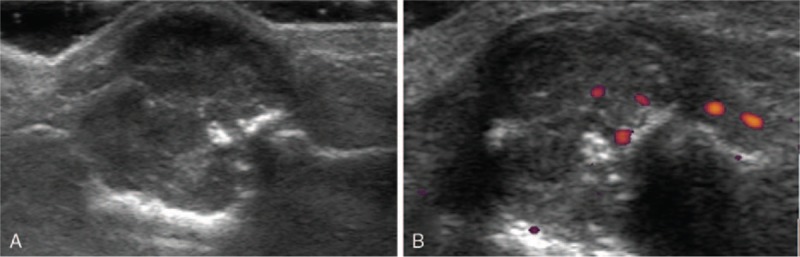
Case 2. (A) Two-dimensional ultrasound showed punctuate or patchy calcification in the mass, and discontinuous bone cortex. (B) Point-like blood signal presented in Doppler ultrasound.
